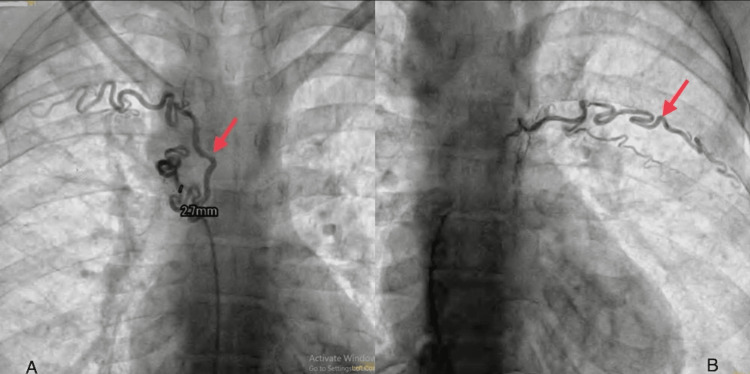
(A) Computed tomography pulmonary angiography showing a 2.7 mm MAPCA in the peri-tracheal area (marked by the red arrow); (B) angiogram showing MAPCA supplying the upper lung lobe (marked by the red arrow) The images were taken by the authors MAPCA: multiple aortopulmonary collateral artery
